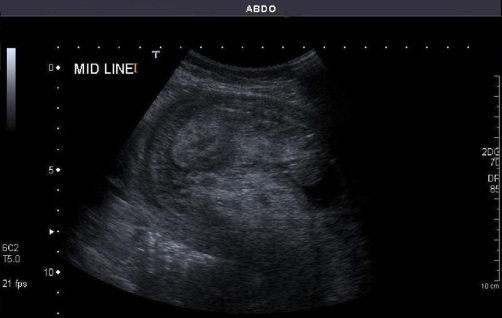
Ultrasound image showing features suggestive of intussusception.
